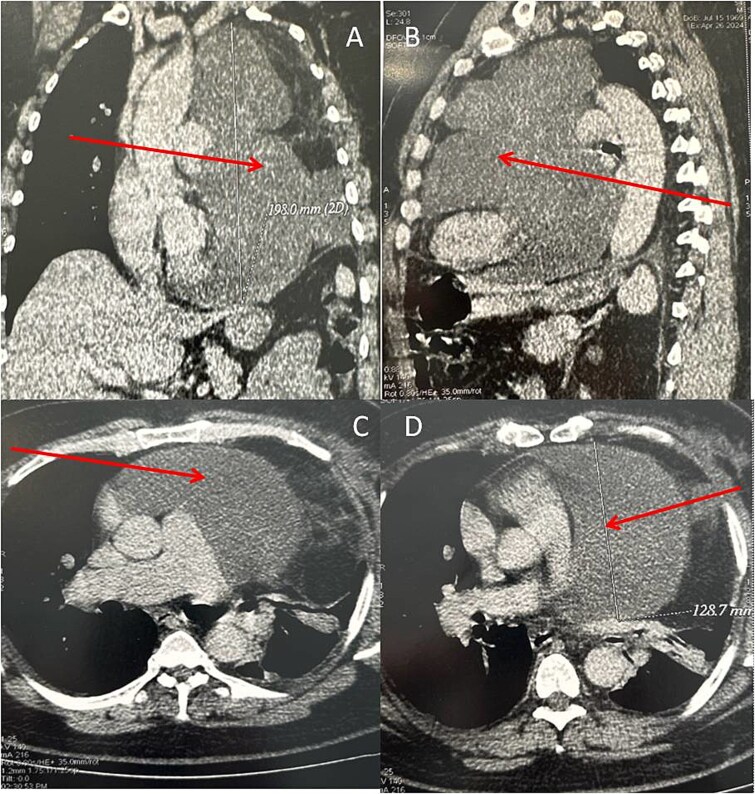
Chest CT scan (A: coronal section, B: sagittal section, C and D: axial section) in spiral mode with injection of contrast agent showing a giant pericardial mass (arrows).
